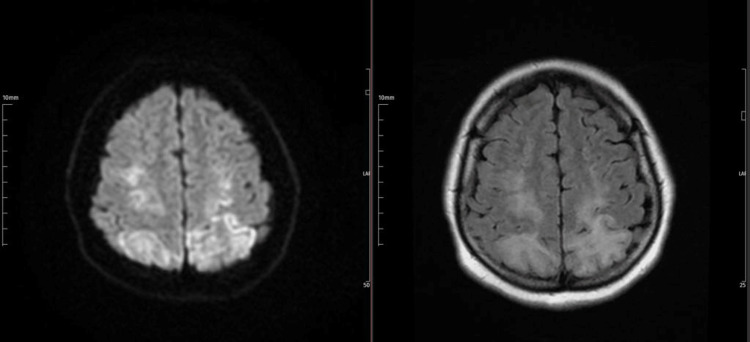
Axial diffusion-weighted magnetic resonance imaging showed prominent bilateral hyperintensity on the posterior regions of the brain's left side consistent with posterior reversible encephalopathy.
